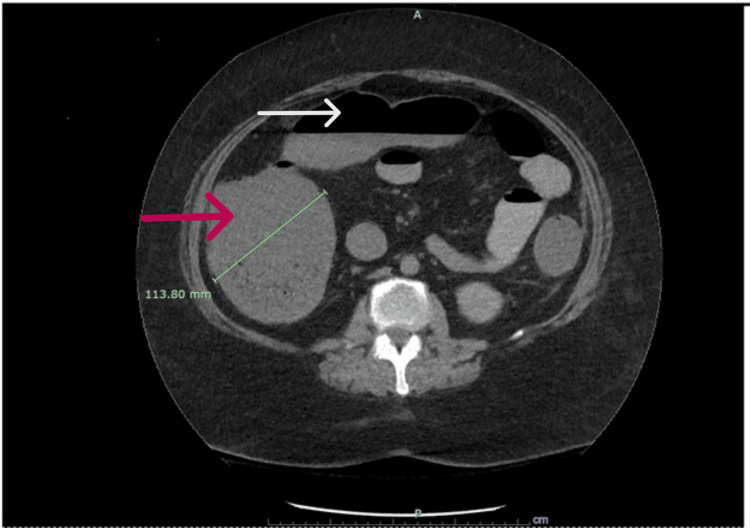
Cross-sectional image of CT of the abdomen without contrast. The white arrow is pointing toward the air fluid levels in the colon, suggesting a high small bowel obstruction. On the left side, the red arrow is pointing toward a severely distended colon measuring 11.3 cm, filled with stool.
